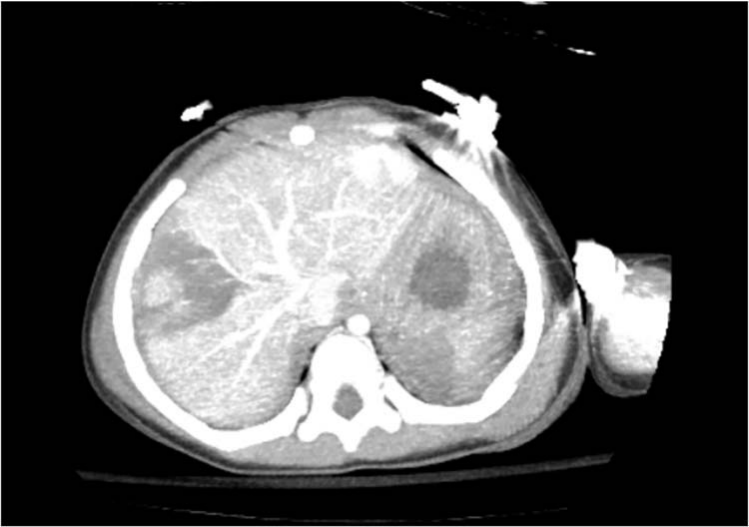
Axial view of CT image revealing liver and splenic injuries.
